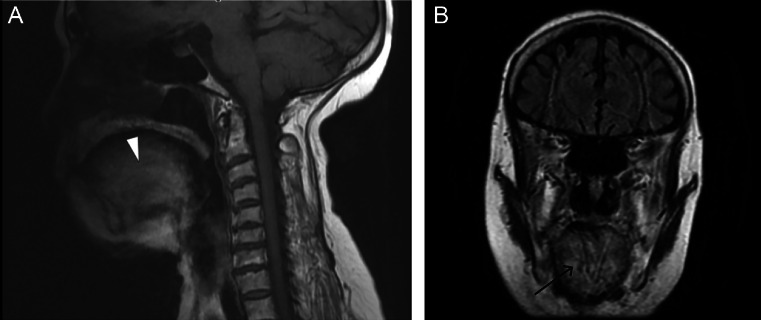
(A) Mid-sagittal T1WI brain MRI demonstrates diffuse hyperintense signal within the tongue musculature (White arrowhead). (B) Coronal FLAIR brain MRI at the level of the mid-tongue shows diffuse, symmetrical hyperintense signal within the intrinsic tongue muscles. (Black arrow).
